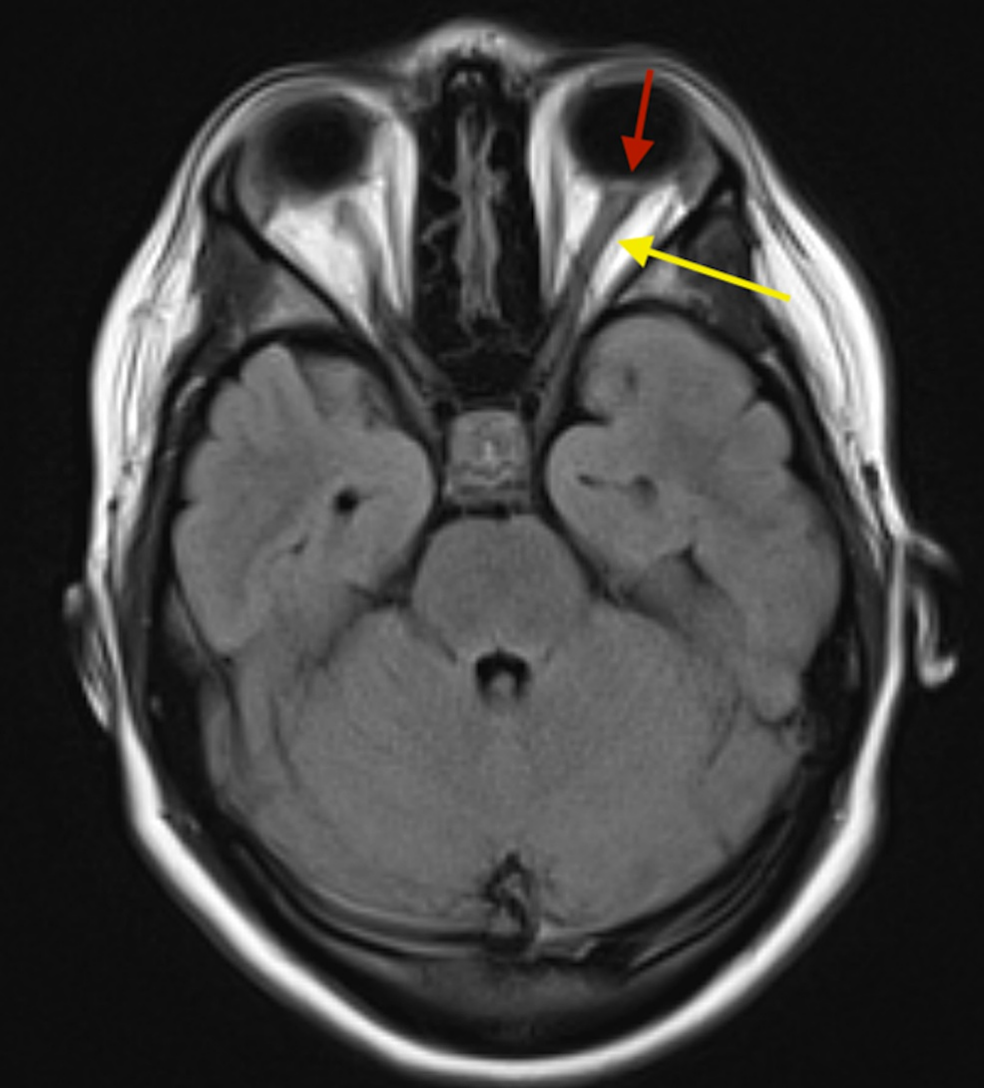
FLAIR MRI of the brain (axial section) showing thickened optic nerve sheath (yellow arrow) and flattened lamina cribrosa of sclera (red arrow). FLAIR MRI: Fluid-attenuated inversion recovery magnetic resonance imaging
